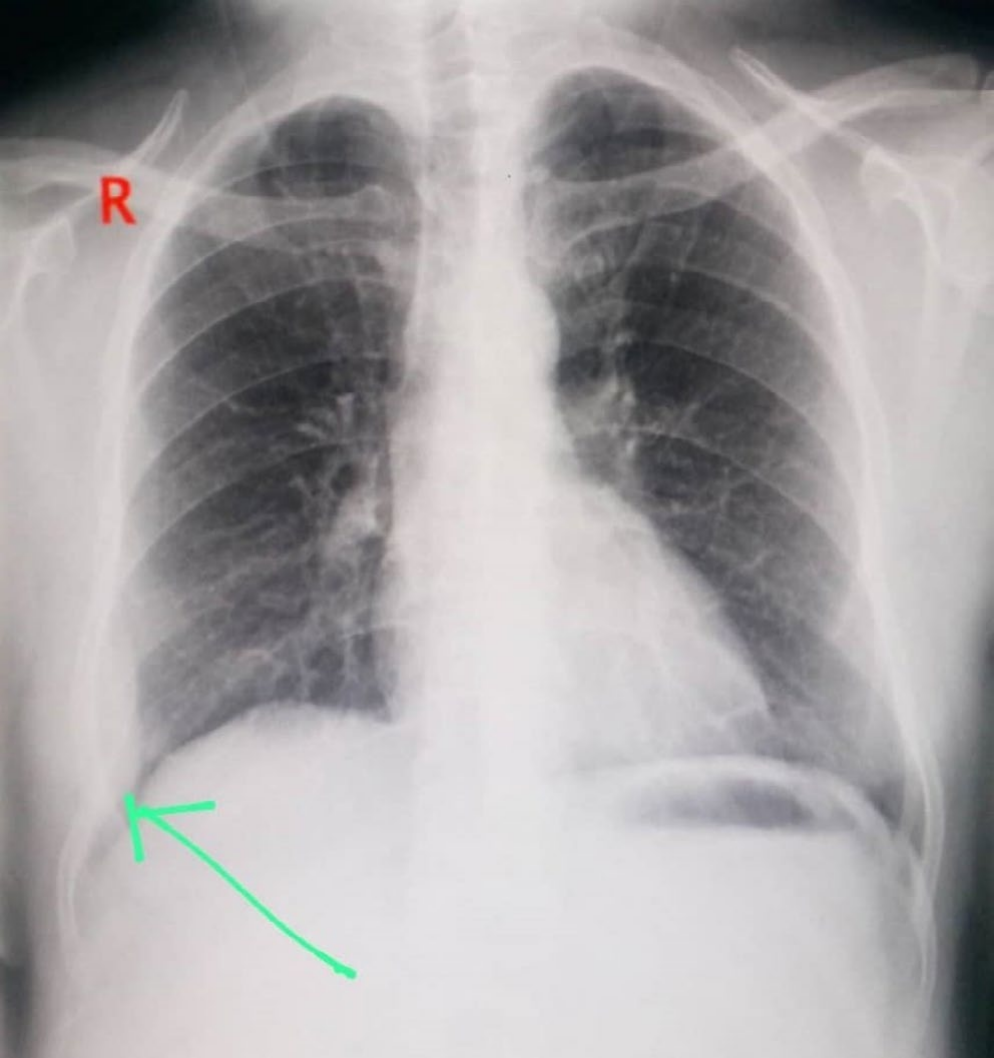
Posteroanterior chest radiograph. A pleural‐based opacity projects over the right lower hemithorax along the lateral chest wall (green arrow), suspicious for a rib‐origin lesion. Cardiomediastinal contours are preserved; no focal parenchymal consolidation is seen.
